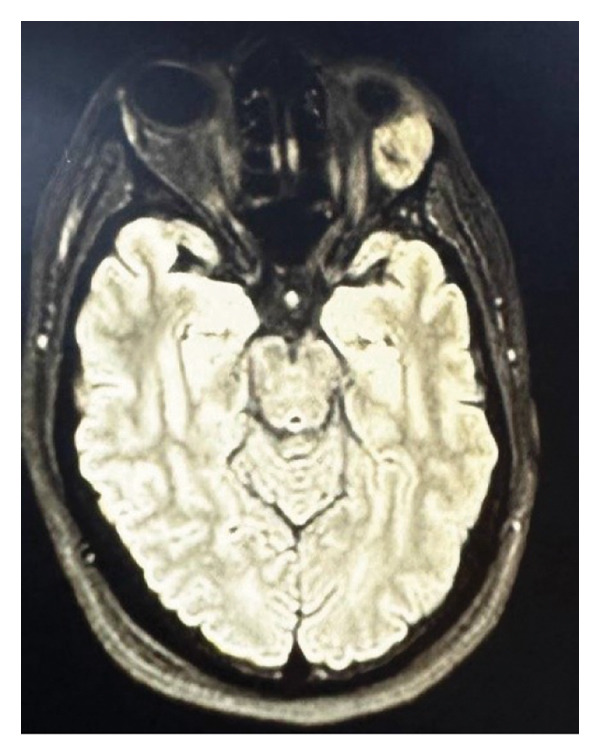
Transverse MRI showing a well‐defined orbital mass in the superior extraconal compartment of the left orbit.
